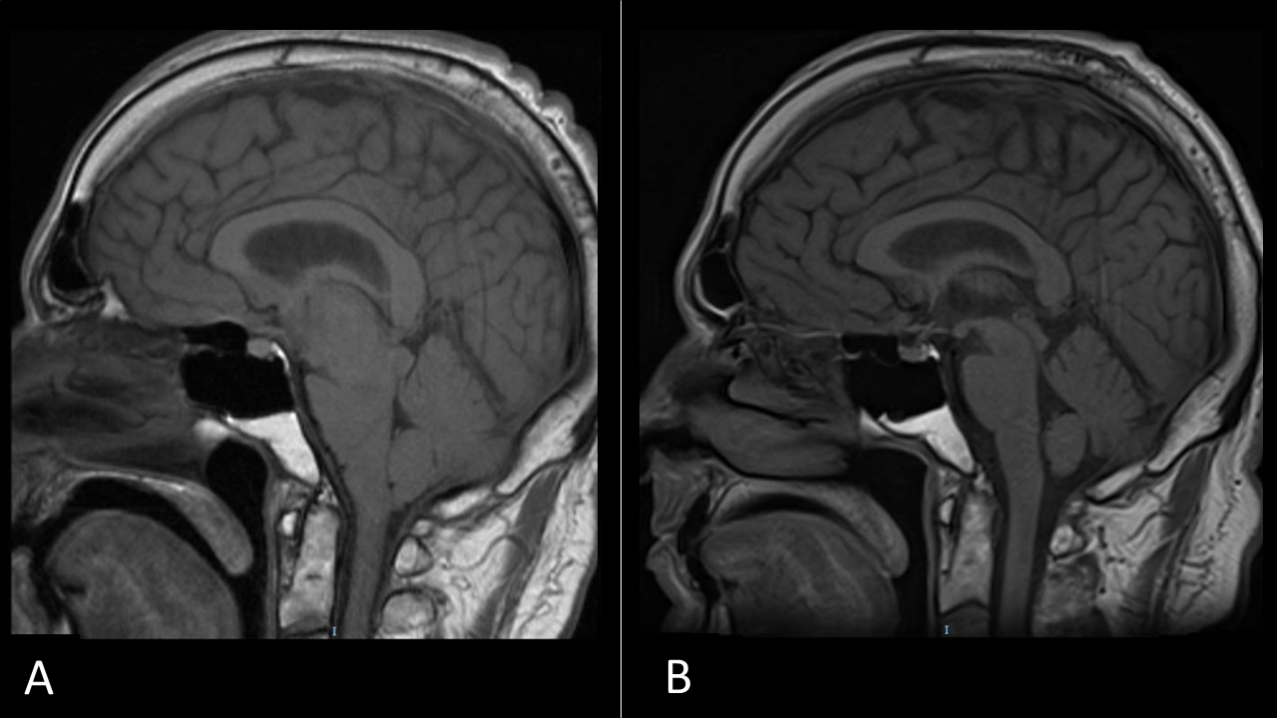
Intracranial Hypotension Before and After Dural Repair T1-weighted sagittal MRI nine months after surgical resection (A) showing brain stem sagging, flattening of the pons, decreased pontomesencephalic angle, decreased mamillopontine distance, and mild uncal herniation consistent with intracranial hypotension and (B) resolution of these findings six weeks after blood and fibrin glue patching.
